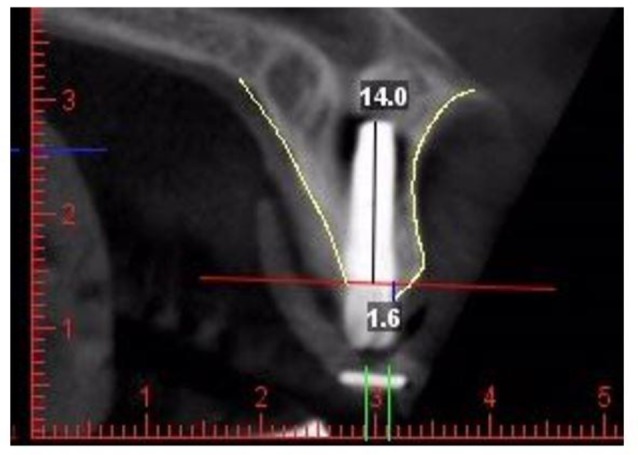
Measurements of bone height from crestal point of the buccal bone plate to the platform of the implant on the midbuccal cross-sectional cone beam computed tomography slice after a straight line was drawn in the direction of the long axis of implant and another in the platform region
